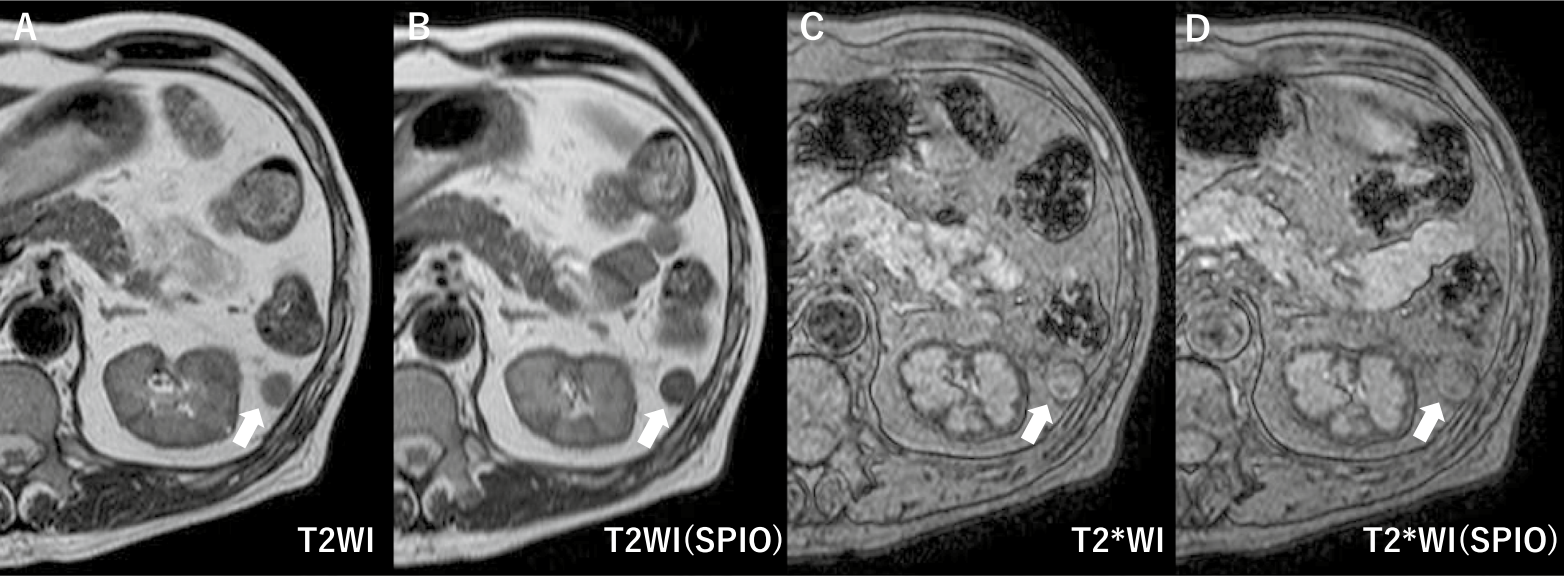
Accessory spleen imaging via SPIO-enhanced MRI: the MRI highlighted a marked signal reduction (arrow) on the SPIO-enhanced T2 and T2* weighted images (WI). A T2WI, B SPIO-enhanced T2WI, C T2*WI, D: SPIO-enhanced T2*WI
